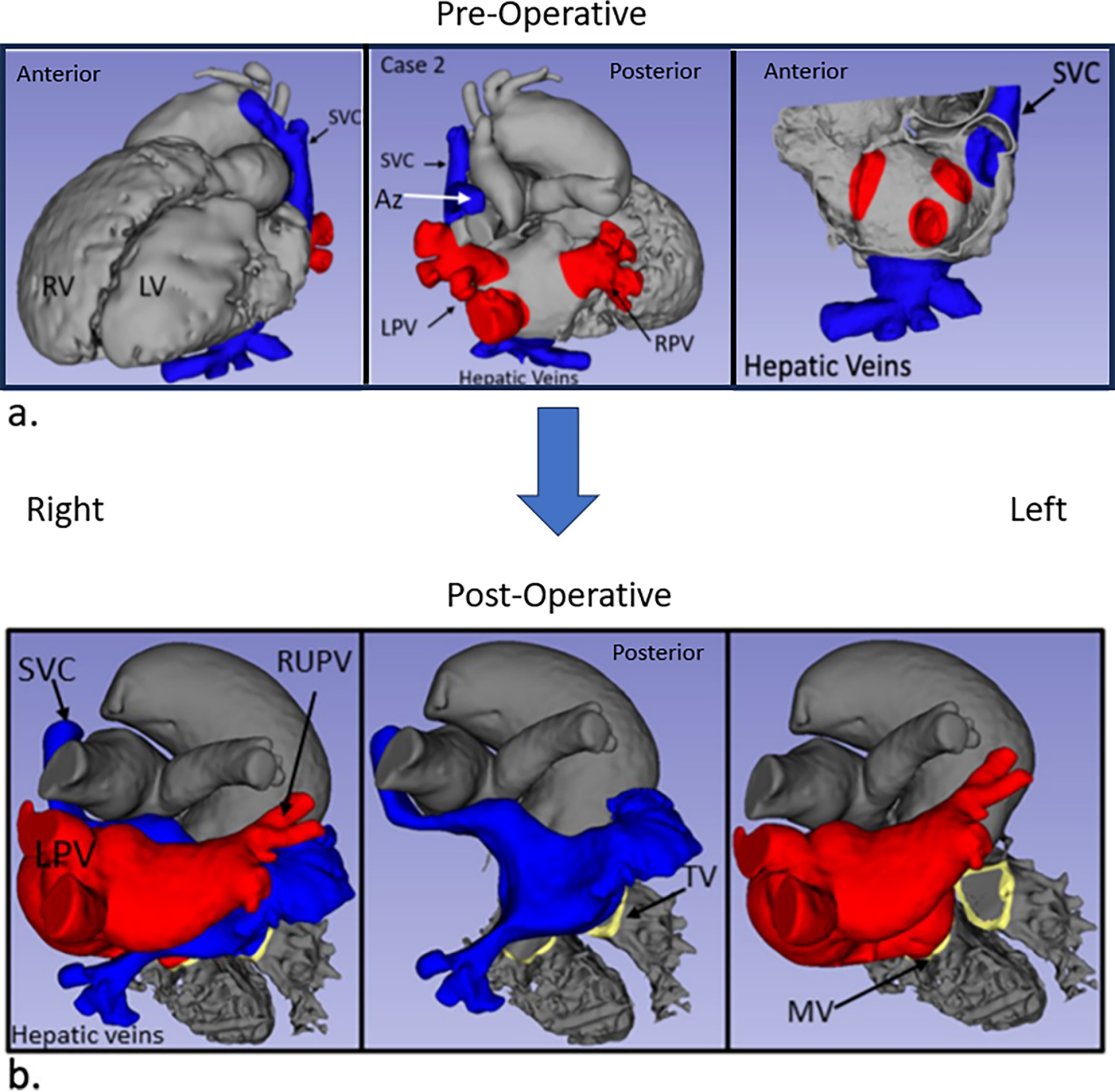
Case 2 imaging derived from CT. Pre-operative (a) and post-operative (b) images are shown with comparable views to Figures 1a,b.
